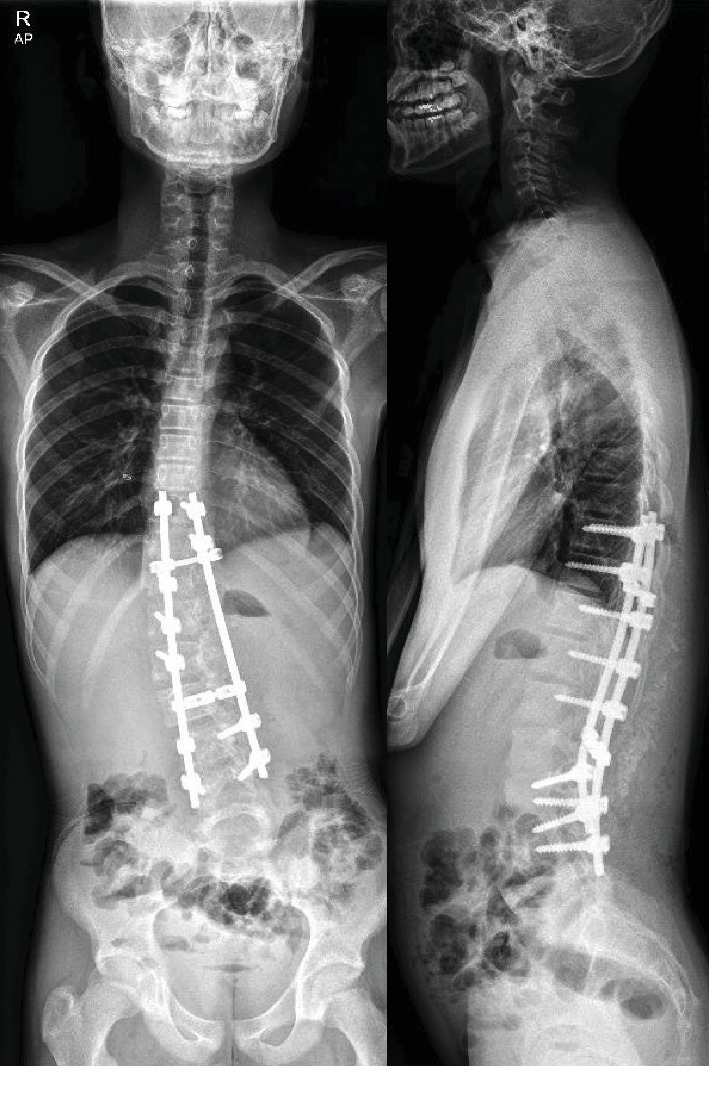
Whole spine plain radiograph. AP and lateral view at the immediate post-op, January 2^nd^, 2016.
